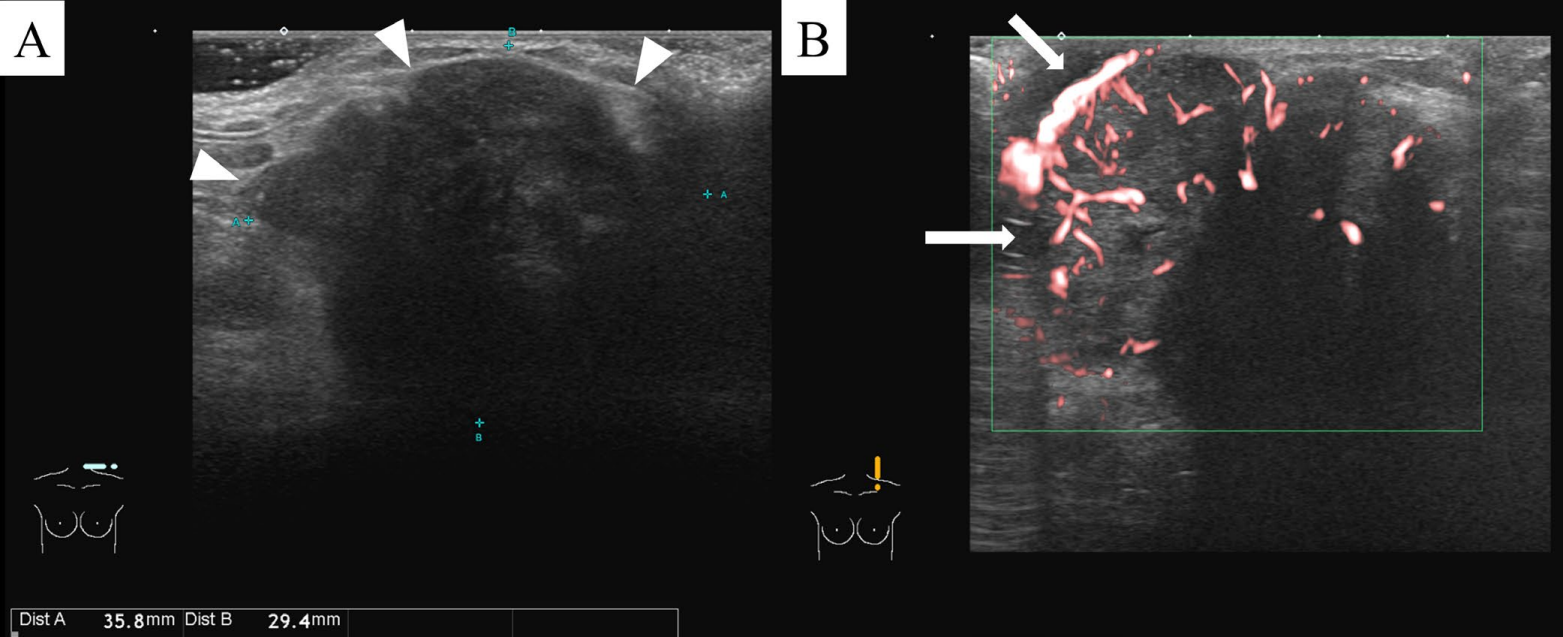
Ultrasonography images of the mass in the left supraclavicular region (A: B‐mode, B: Power Doppler mode). A hypoechoic mass with irregular margins and a lobulated, heterogeneous internal echo pattern measuring 36 × 29 mm is observed (A, arrow heads). Increased blood flow signals are observed within the mass in power Doppler mode (B, arrows).
